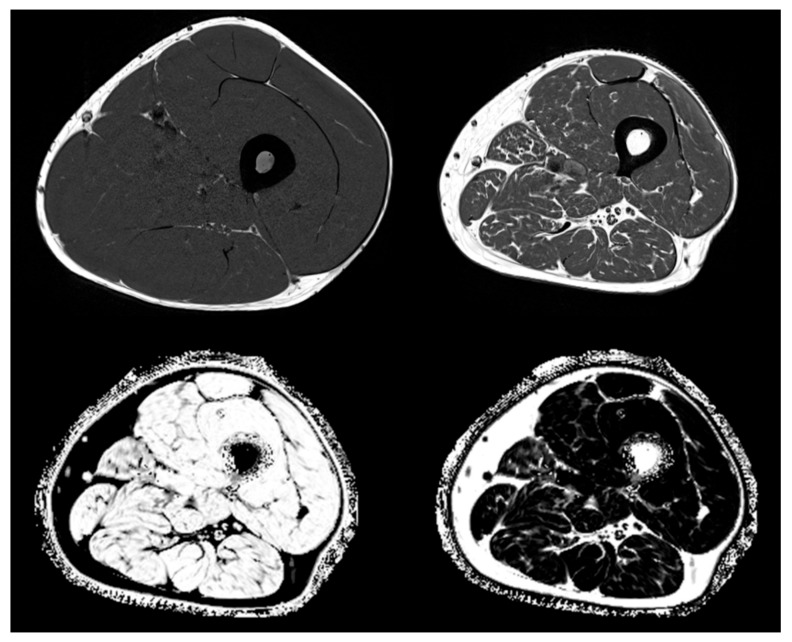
Magnetic resonance imaging (MRI)-based muscle imaging. Weighted image of young healthy (top left) and elderly sarcopenic male (top right). Water fraction (bottom left) and fat fraction (bottom right) images of subject shown in top right.
